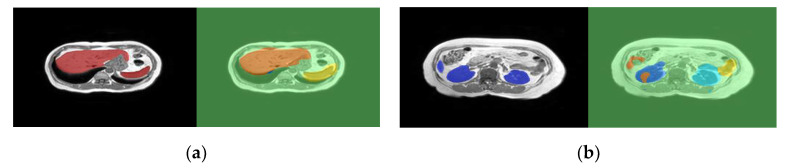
Example magnetic resonance image (MRI) segmentation of independent test images using DeepLabv3 segmentation network. The left of each image is the groundtruth on a black background, the right is the segmentation: (a) is a slice showing the liver and spleen; (b) is another slice showing the kidneys and a small extremity of the liver.
